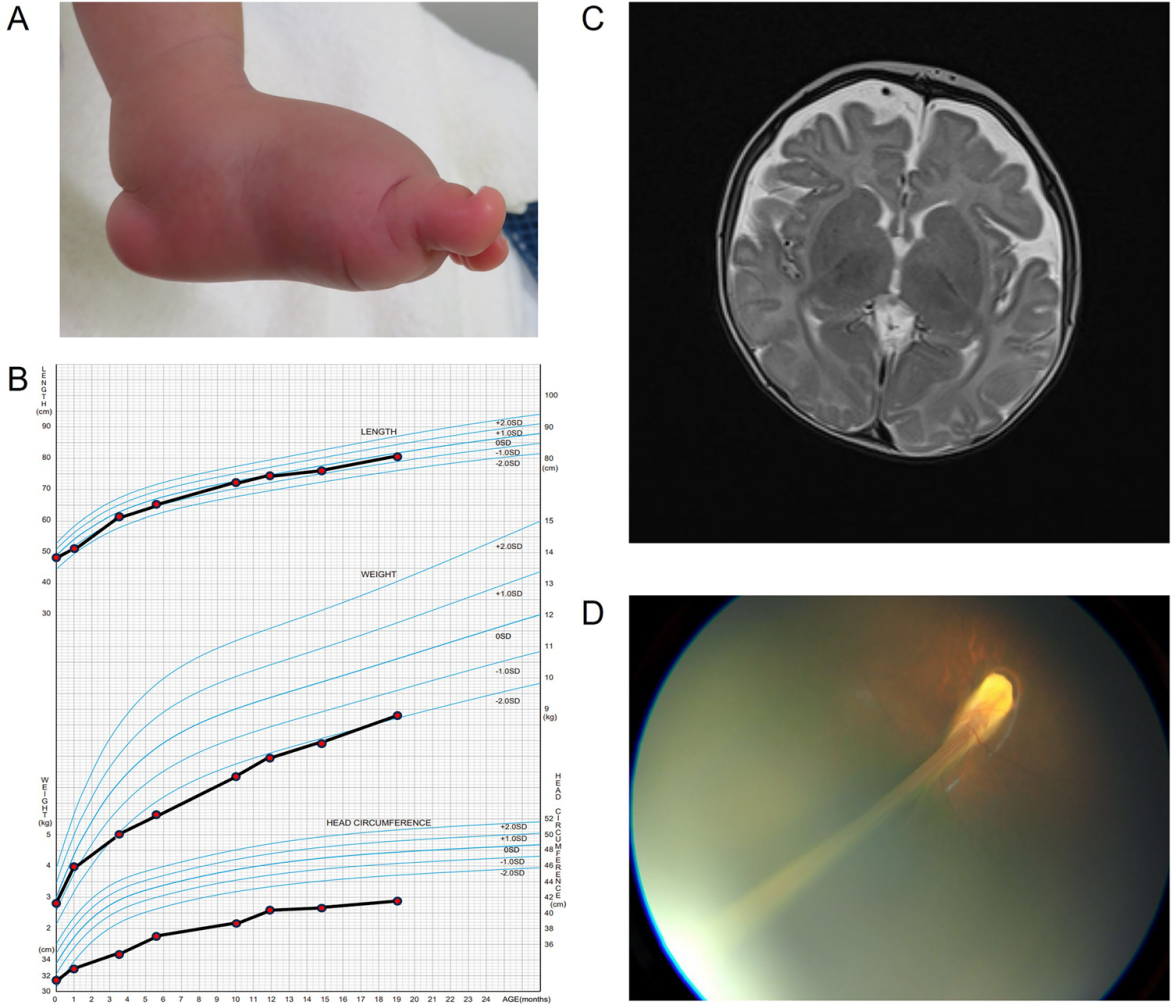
Clinical features of the patient. A Lymphedema on the feet at 1 month of age. B Cross-sectional growth chart for age^9,10^. C Head MRI findings at 3 months of age. D Retinal fold shown by fundus photograph at 5 months of age.
